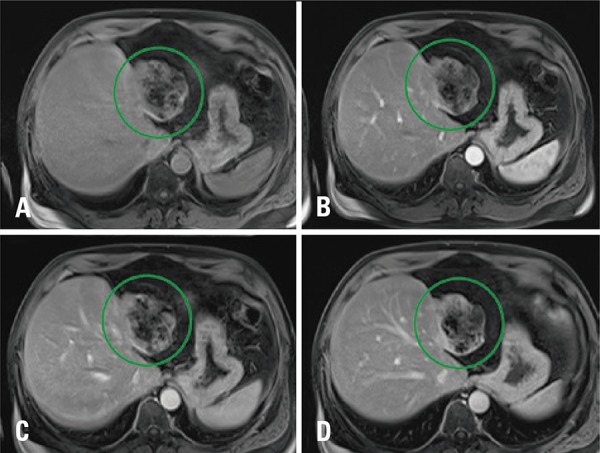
Evolution of hyalinized hemangioma. Axial sequences of the magnetic resonance in T1-weighted image pre- (A) and post-contrast arterial (B), portal (C) and delayed (D) phases, showing volumetric reduction and heterogeneous enhancement pattern
